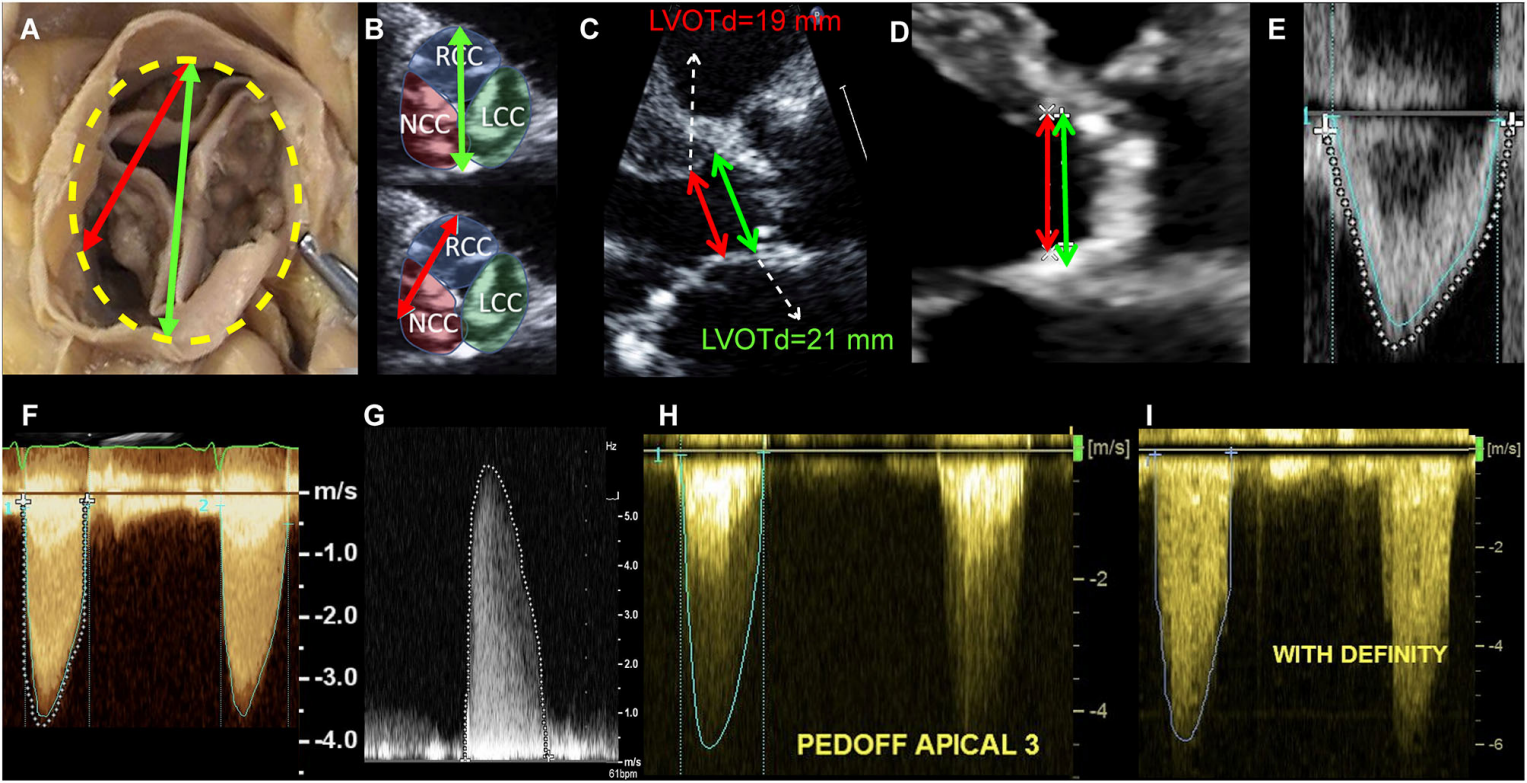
Echocardiographic measurement pitfalls and how to avoid them. LVOT diameter must be measured at its maximal dimension, which generally corresponds to the bisection between the right coronary cusp hinge point anteriorly and the interleaflet triangle between the left and non-coronary cusps posteriorly (A,B). LVOTd must be measured at the annulus and not 5–10 mm below, as this leads to significant underestimation of AVA and SV (C). In this case, measuring 5 mm below the annulus (as recommended in guidelines) lead to an LVOT area of 2.83 cm^2^, as compared to an area of 3.46 cm^2^ when measured at the annulus (18% underestimation, therefore leading to significant underestimation of SV and AVA). In case of LVOT ectopic calcification, if the plane that bisects the largest diameter cannot exclude the calcium, LVOT diameter measurement should include the calcium in the measurement (D). LVOT velocity-time integral should be measured at the modal velocity (the densest line of the Pulse Wave Doppler) since flow at the LVOT is laminar (E: blue trace represents the modal velocity whereas the dashed white line overestimates LVOT VTI). For an accurate measurement of the transaortic jet velocity, tracing should be done at peak velocities but excluding fine linear signals (F: green trace represents the correct measurement, whereas the dashed white line overestimates aortic valve VTI by including linear signals). It is paramount that the Doppler beam is optimally aligned parallel to the stenotic aortic jet. Therefore, a meticulous search of the highest transvalvular velocity is mandatory. This requires a comprehensive Doppler study that is not only limited to the apical window but also includes right parasternal, suprasternal, and sometimes subcostal approaches using a small, dedicated CW Doppler transducer (*pencil probe* or *Pedoff transducer*) (G,H). Finally, the use of ultrasound enhancing agents (i.e., contrast echocardiography as with Definity®) might lead to overestimation of transvalvular velocities and gradients and therefore caution should be taken (I).
